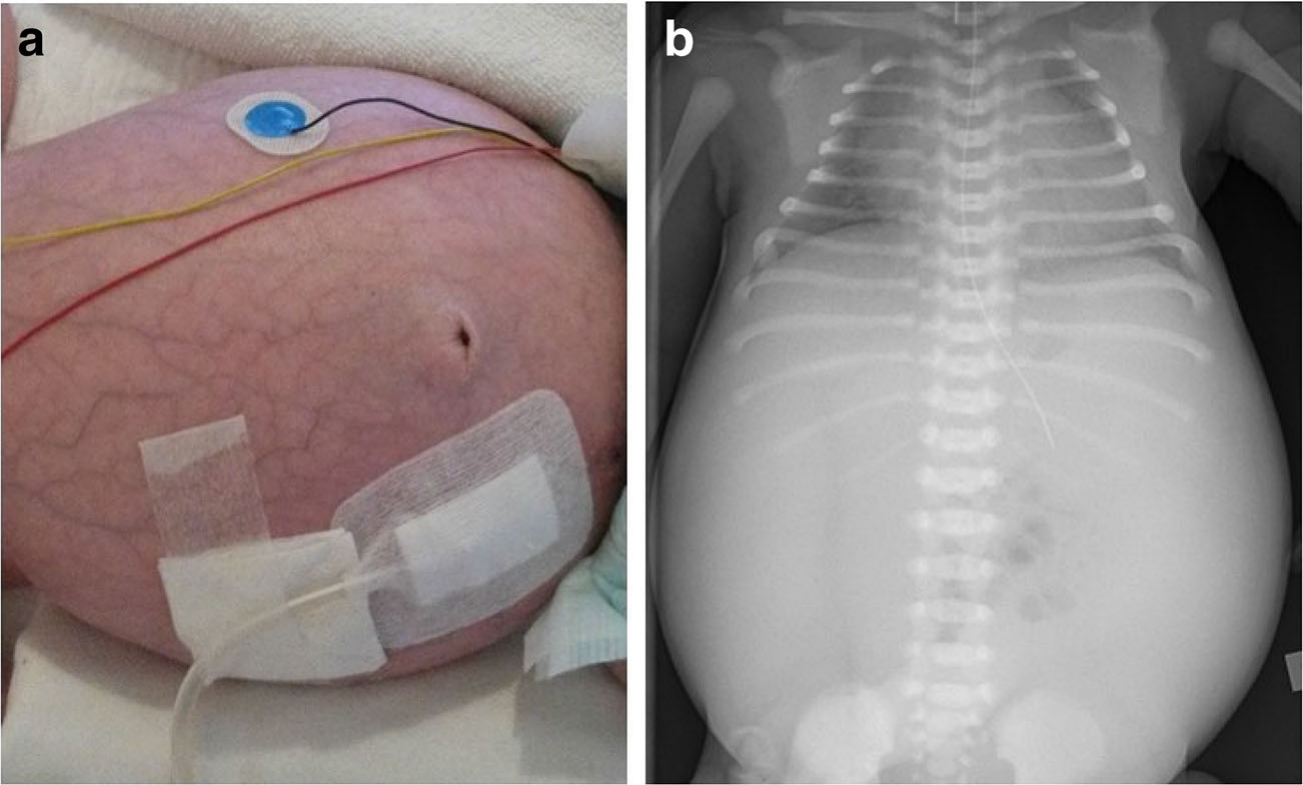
Clinical presentations of ARPKD with an enlarged abdomen (a) or pulmonary hypoplasia on chest radiography (b)
